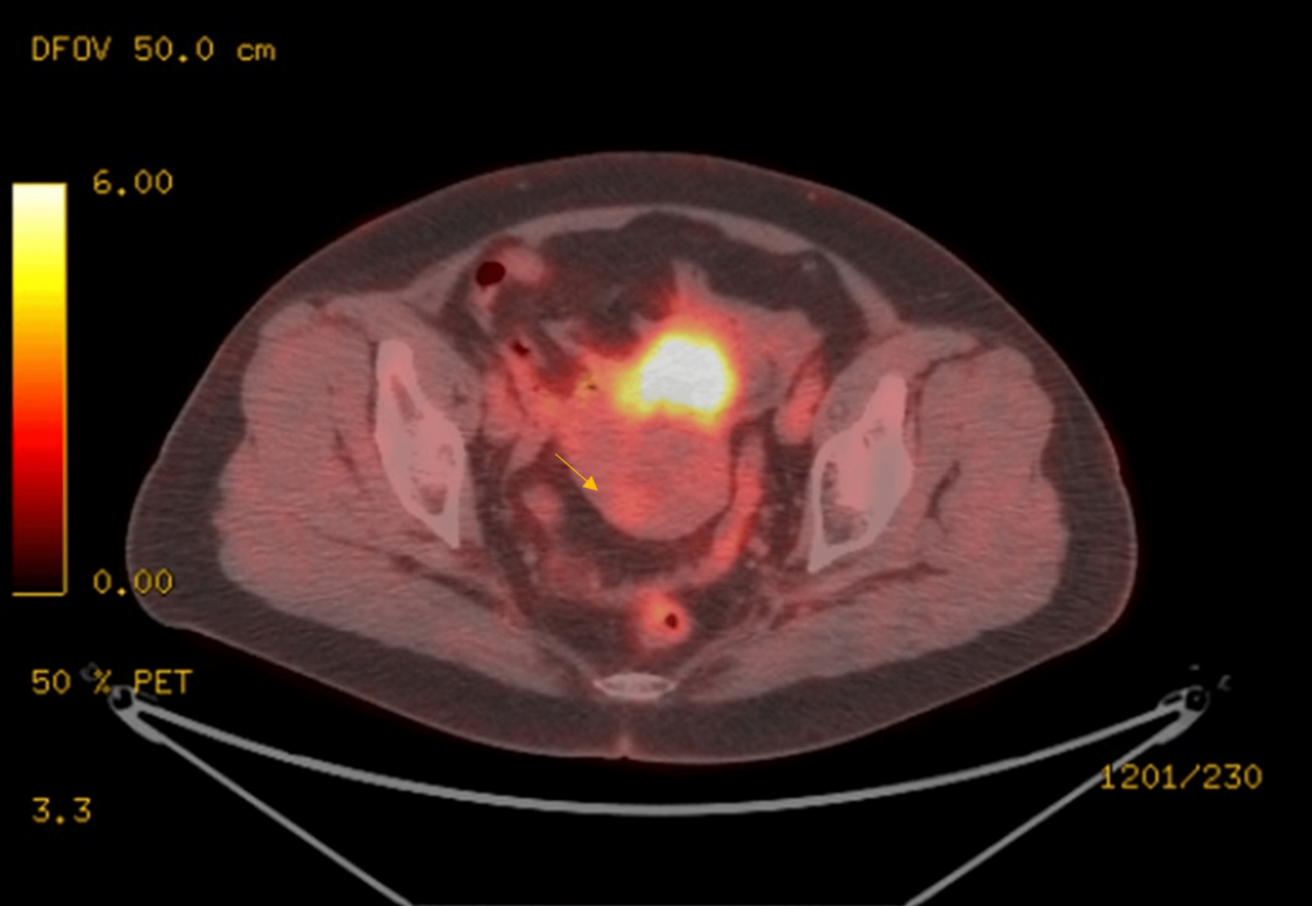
FDG-PET/CT A non-significant FDG uptake was observed in the cyst’s solid component (indicated by the arrow). FDG-PET, 18F-fluoro-deoxyglucose positron emission tomography; CT, computed tomography
